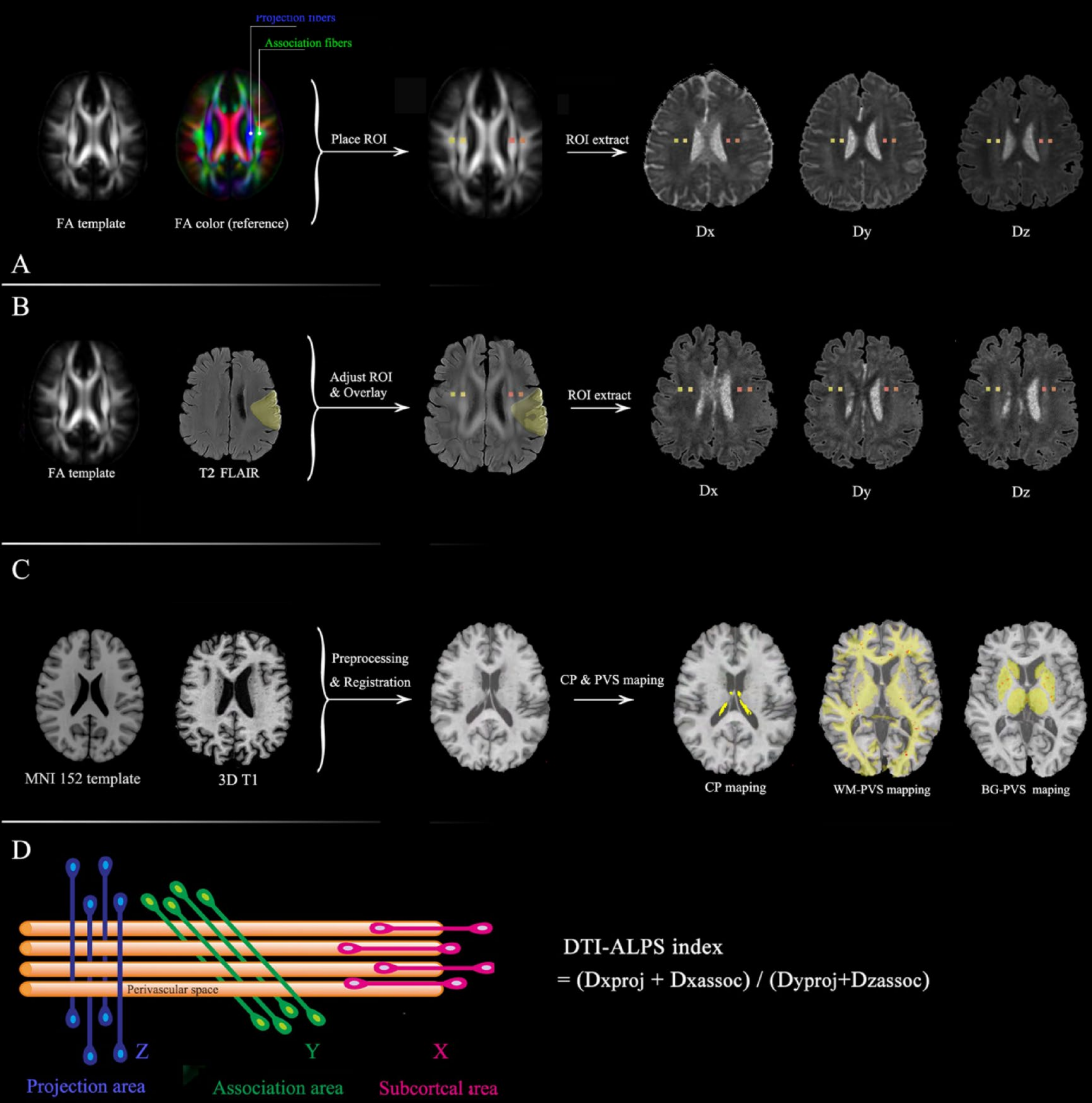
The assessment process for the glymphatic function by calculating the DTI-ALPS index in non-stroke cohorts (A) and stroke cohort (B), and CP and PVS volume (C). The goal for the placement of the ROIs (yellow and orange rectangles) is to place them on their bilateral projection fibers (blue area) and association fibers (green area), respectively (D) in calculating DTI-ALPS index. The relationship between the direction of the perivascular space (orange cylinder) and the direction of the fibers. ALPS, diffusion tensor image analysis along the perivascular space; FA, fractional anisotropy; FLAIR, fluid-attenuated inversion recovery; ROI, region of interest; MNI, Mapping-Montreal Neurological Institute; CP, choroid plexus; PVS, perivascular space; WM, white matter; BG, basal ganglia.
